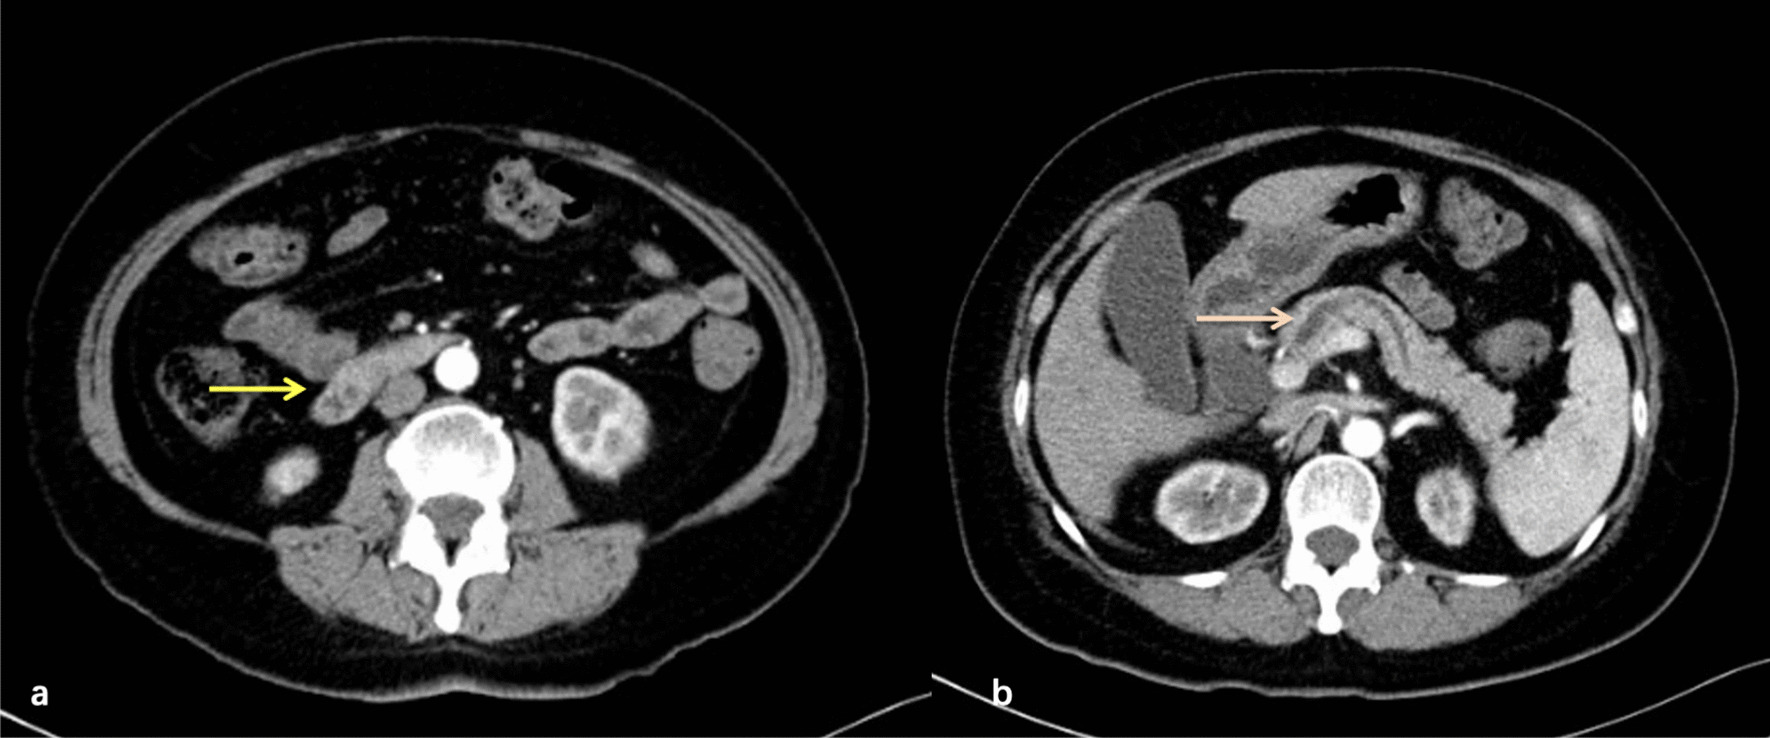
Contrast-enhanced CT 6 months ago found suspicious nodules at the duodenal papilla (a) and dilatation of pancreatic duct (b)
